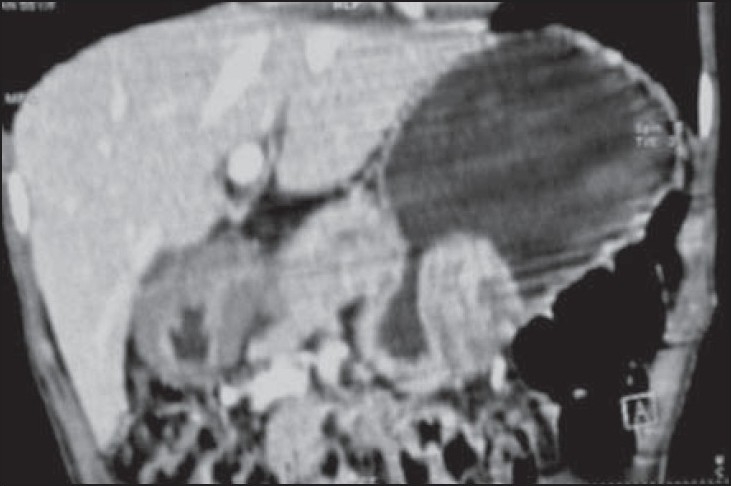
Oblique coronal CT MPR image showing polypoidal neurofibroma along the greater curvature of the stomach along with diffuse thickening of the wall of the pyloric antrum
